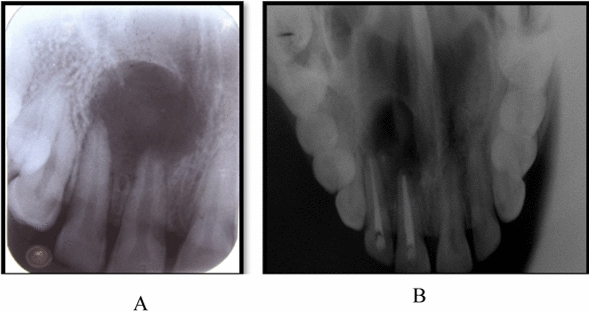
A Periapical radiolucency was seen on intraoral periapical radiograph associated with regions 11 and 12. B Periapical radiolucency was seen on occlusal radiograph associated with regions 11 and 12
